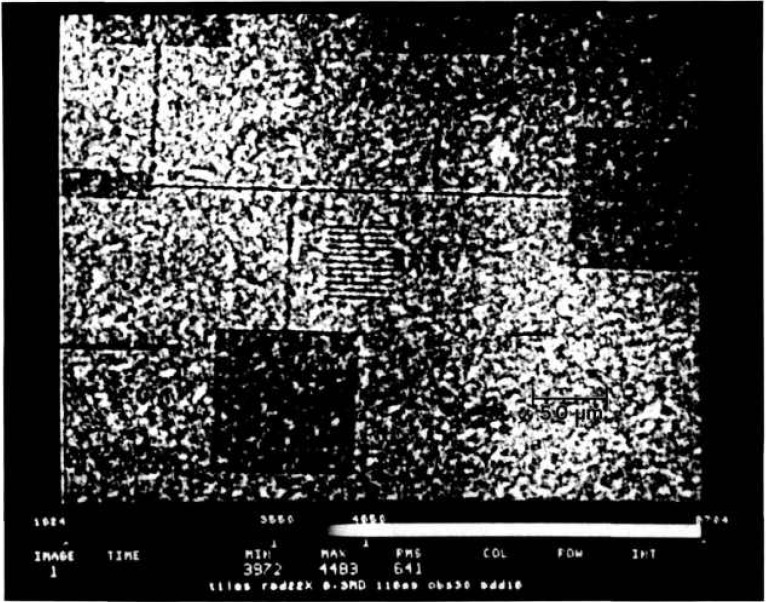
A radiographic image of another section of Pd pattern with a magnification of 22 × at 8.3 keV.
